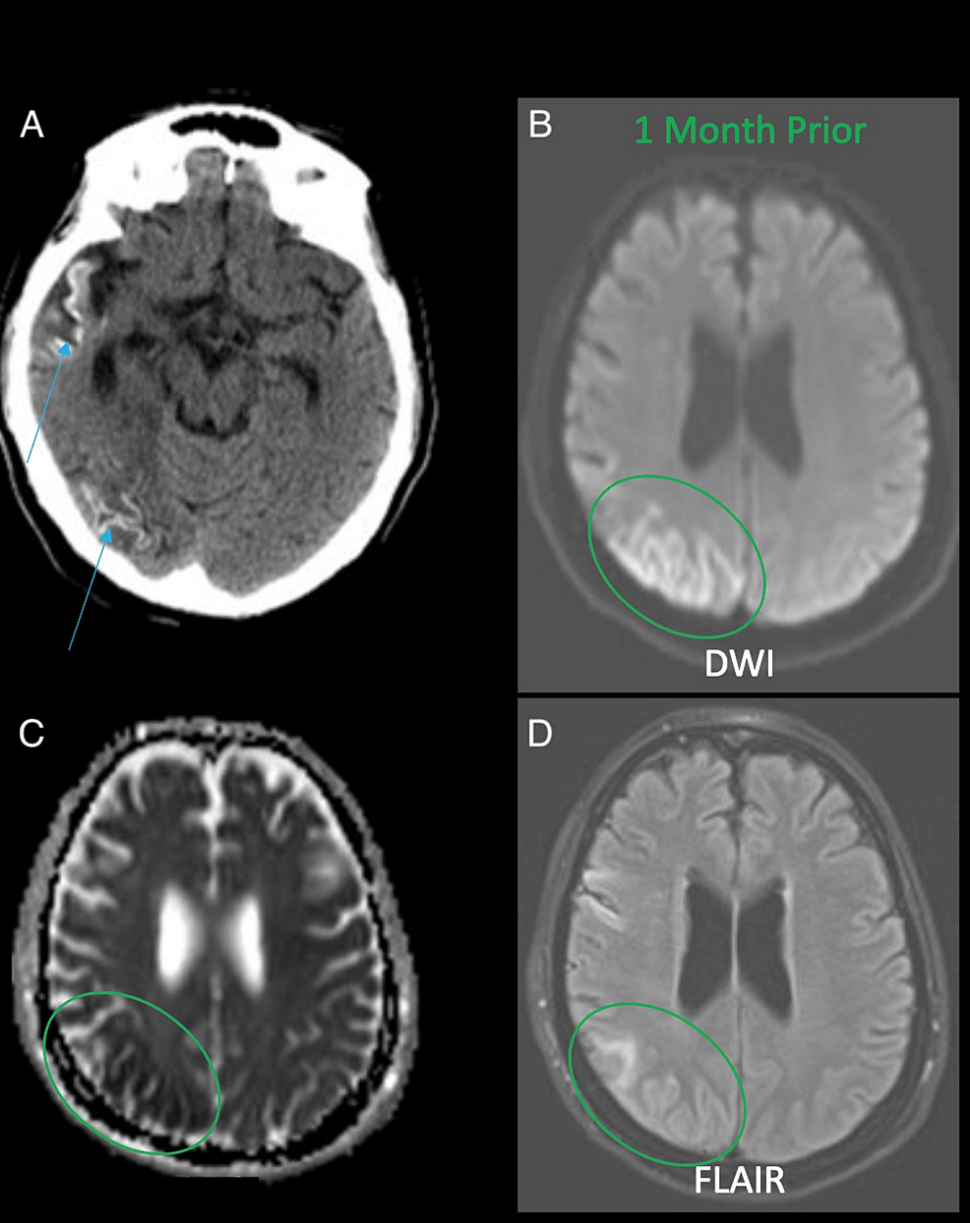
Two sets of images, CT (left) and MRI (right) in a patient one month apart demonstrating cortical laminar necrosis. Panel A shows areas of increased laminar density secondary to cortical laminar necrosis (blue arrow). Panel C highlights the same area of laminar calcification (green circle). Panels B and D demonstrate an area of decreased diffusion with increased DWI and decreased ADC indicating an acute infarction, due to cortical laminar necrosis (green circle). DWI: diffusion-weighted image, ADC: apparent diffusion coefficient
